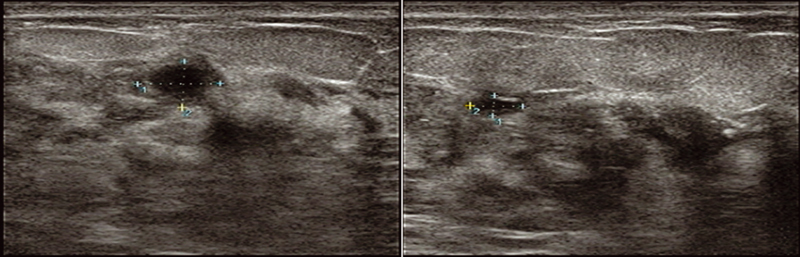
Ultrasound showing small hypoechoic collections.
